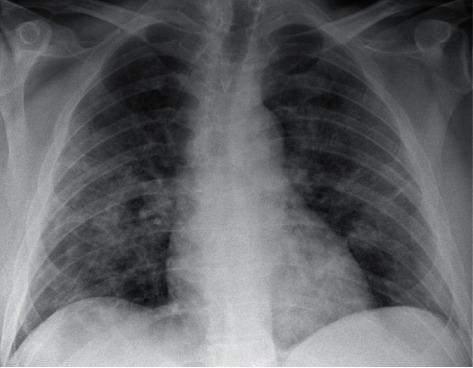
Anteroposterior chest radiographs of the patient during COVID-19 infection approximately 1 month prior to the current hospital admission.
